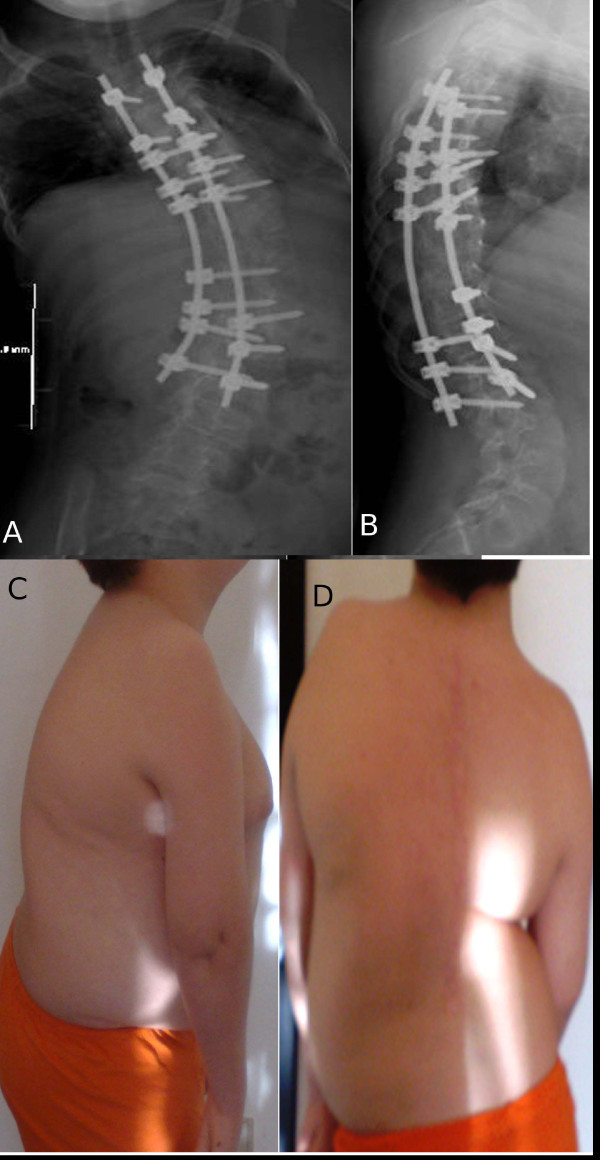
(A, B, C, D), Case 5: Postoperative radiographs, anteroposterior (A) and laterolateral (B) view and clinical picture at a 2-year follow-up (C and D).
